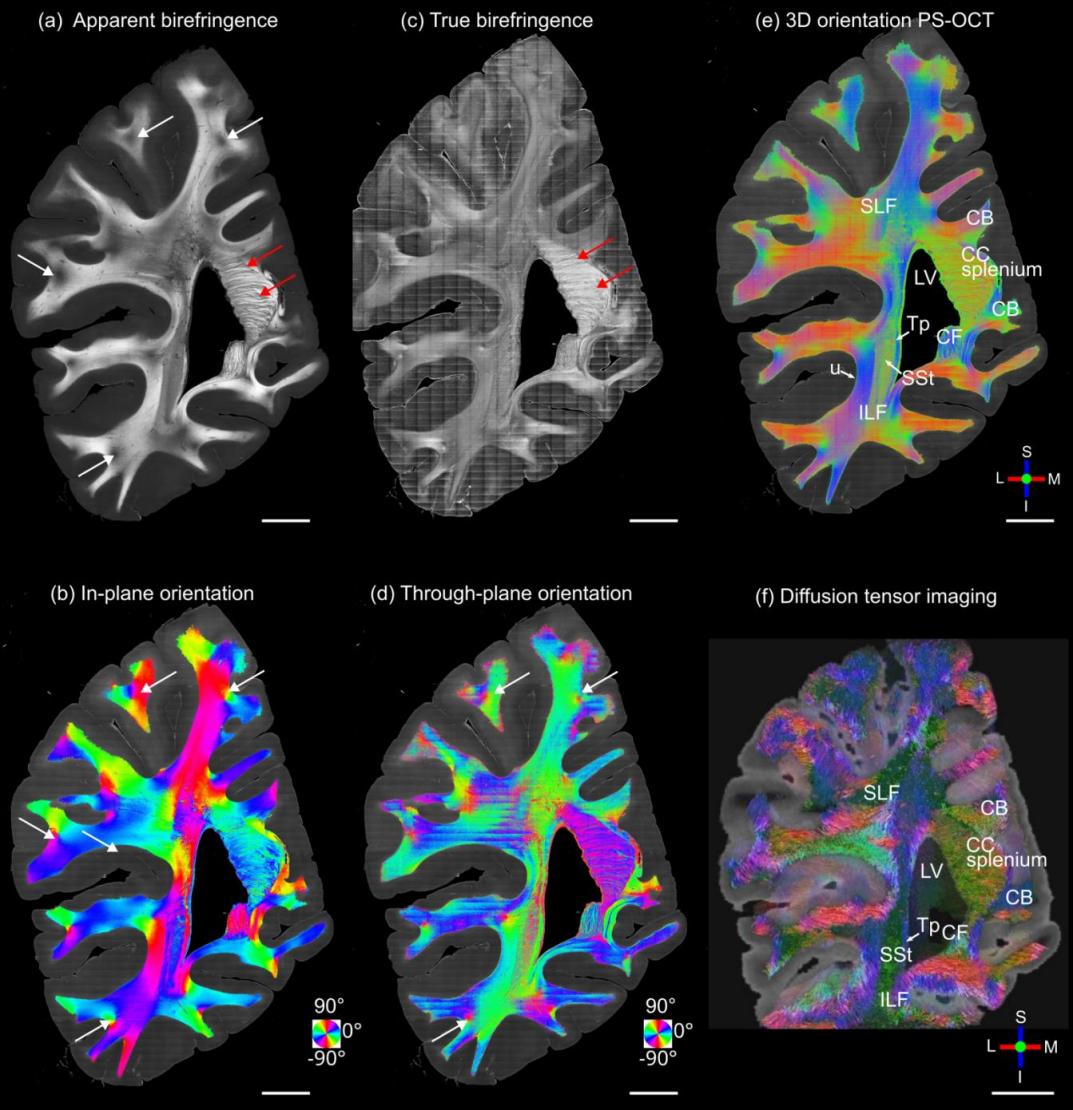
3D fiber orientation maps of the coronal slice sample: (a) apparent birefringence, (b) in-plane orientation, (c) true birefringence, (d) through-plane orientation, (e) 3D orientation from PS-OCT, and (f) 3D orientation from diffusion MRI. Red arrows in (a) and (c) indicate some fibers bundles in the splenium of the corpus callosum. White arrows in (a), (b), and (d) indicated some fibers bundles beneath the sulcus region. The in-plane and through-plane orientations are color-coded as indicated by the color wheels in (b) and (d). 3D orientations are color-coded as indicated by the red (left/right), green (anterior/posterior) and blue (inferior/superior) orthogonal axes in (e) and (f). Scale bars: 10 mm. Several anatomical structures are labeled in (d) and (e): LV, lateral ventricle; CC splenium, splenium of the corpus callosum; Tp, tapetum; SSt, sagittal stratum; ILF, inferior longitudinal fasciculus; u, u-fibers. L: lateral; M: medial; S: superior; I: inferior.
